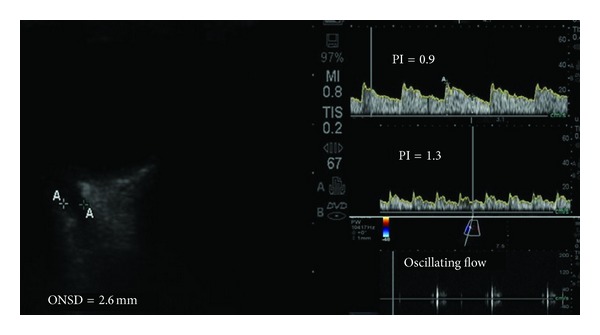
(Left panel) Ultrasound measurements (volume of autologous blood volume infusion = 0.8 mL) by means of the “dark stripe” method depicting an increased ONSD of 2.6 mm in a rabbit with increased ICP (30 mm Hg). (Right panel) TCCD waveforms depicting sequentially: a normal PI (top, volume of autologous blood infusion = 0.1 mL, ICP = 7 mm Hg), an increased PI of 1.3 (middle, volume of autologous blood infusion = 0.6 mL, ICP = 21 mm Hg, and oscillating flow soon after the occurrence of the Cushing reaction (bottom, volume of autologous blood infusion = 1 mL, ICP =40 mm Hg).
